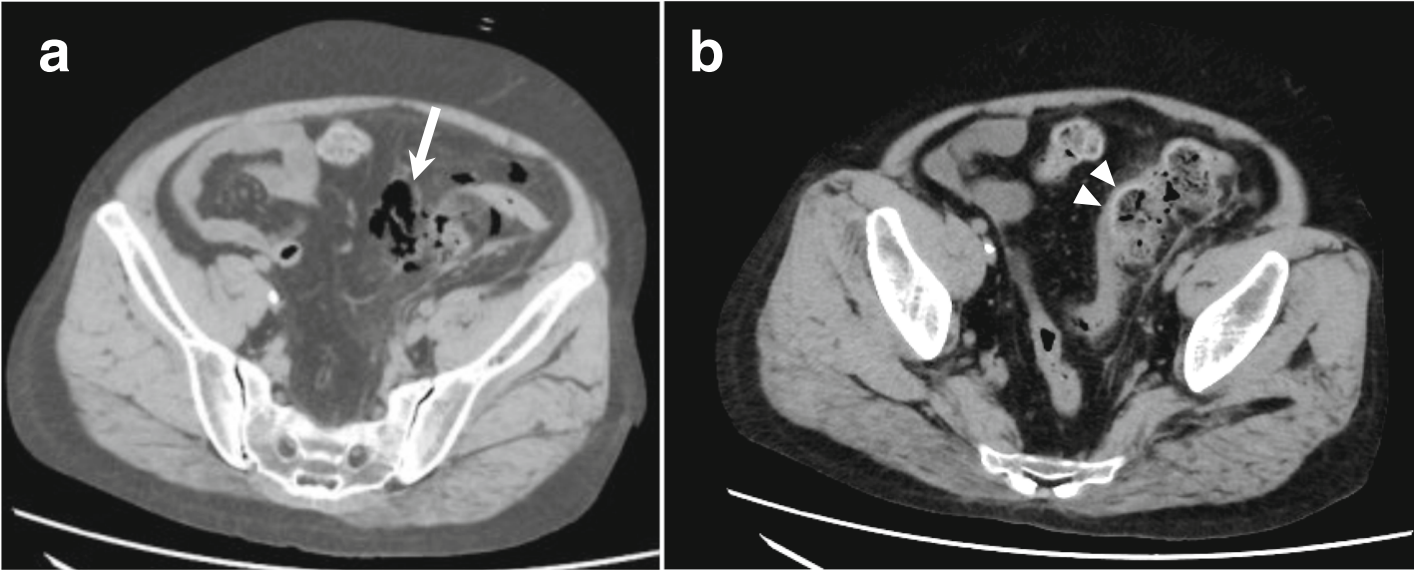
Abdominal contrast-enhanced computed tomography showing a colonic perforation. Mesenteric emphysema (a, arrow) and inflammation (b, arrowhead) of the sigmoid colon are visible
